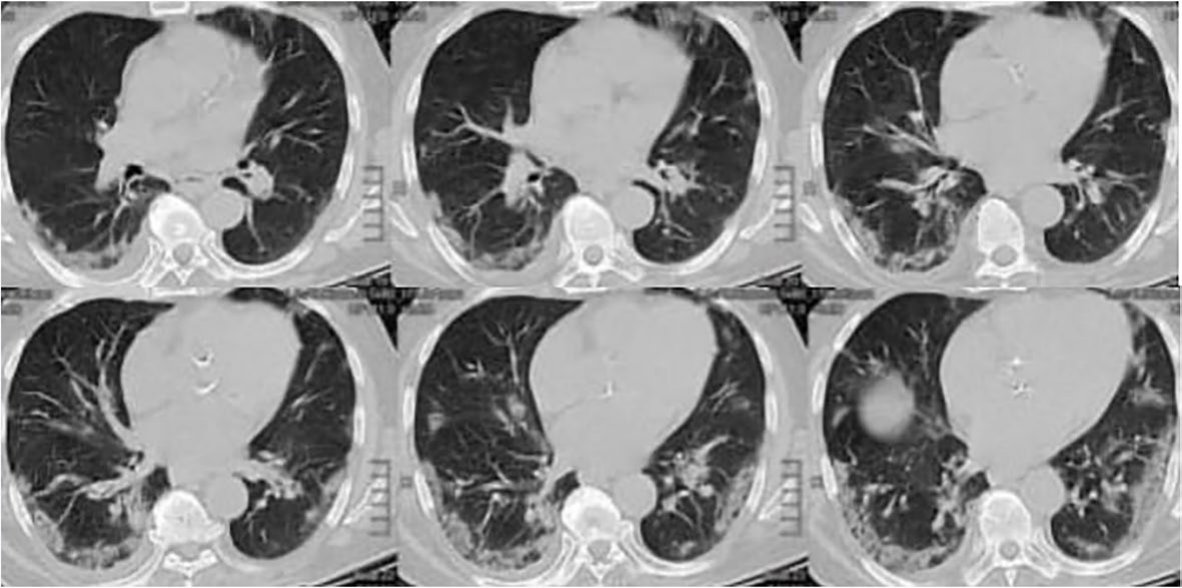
A 62-year-old man restaurant owner was admitted to the emergency room (ER) with a 3-day history of fever (38.2°C/100.8°F) and dyspnea. He reported no other symptoms, nor had a history of travels abroad nor exposure to patients infected or suspected of contagious COVID-19. Xray findings: consolidation with linear brochogram in both lobes us findings: very thick pleural line, Multiple B-lines EF: 40-45% CT findings: GGO and massive consolidation in the posterior parts of the lower lobes. Lymphocytes when he inserted in ICU: 6, 62% troponine at 1st 24h in ICU: 3.2 pg/n. He was confirmed to have Covid19 by PCR
